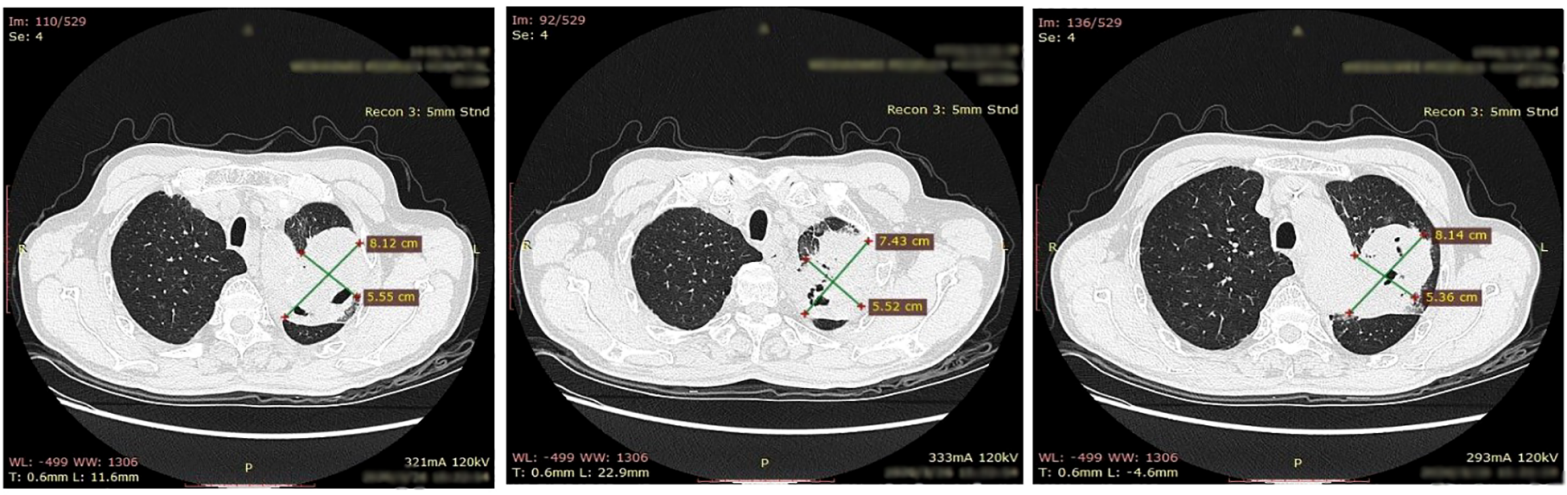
Axial CT images of the upper chest demonstrating an irregular lesion in the left upper lung lobe.
